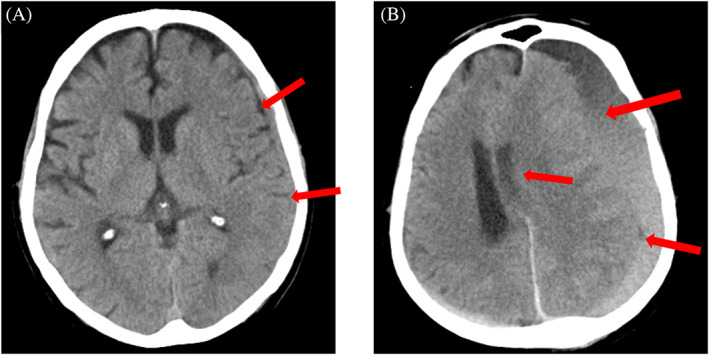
(A) Axial cranial computed tomography (CT) scan obtained upon admission showing subdural hemorrhage (SDH) in the left hemisphere (arrows). (B) Axial cranial CT scan obtained during hospitalization showing massive SDH and midline shift (arrows).
